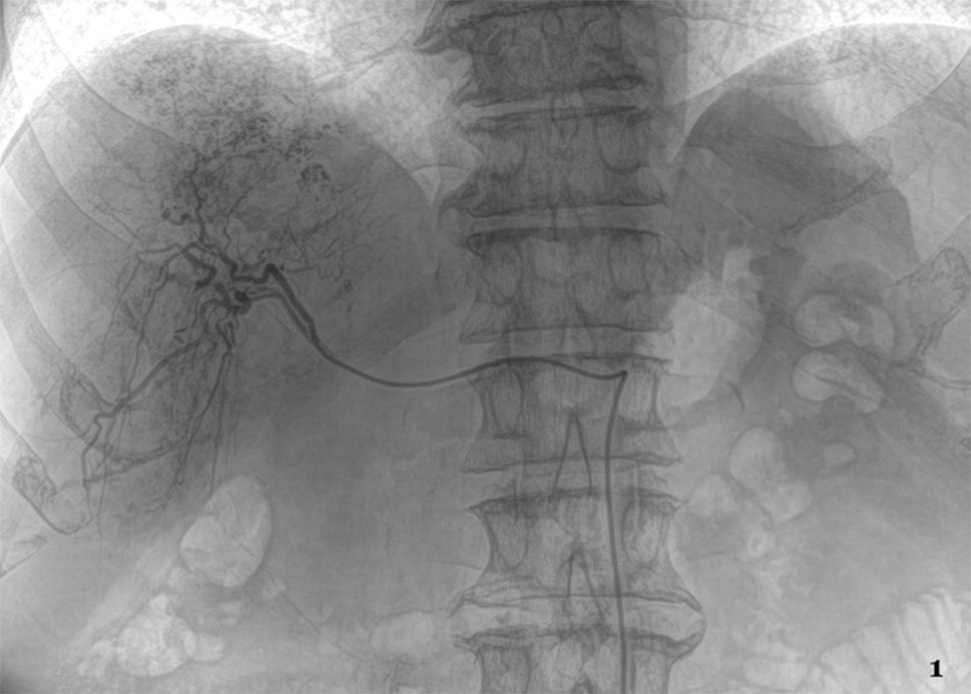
X-ray image. 64 years old male patient with PLC in the RLL during TACE procedure.
